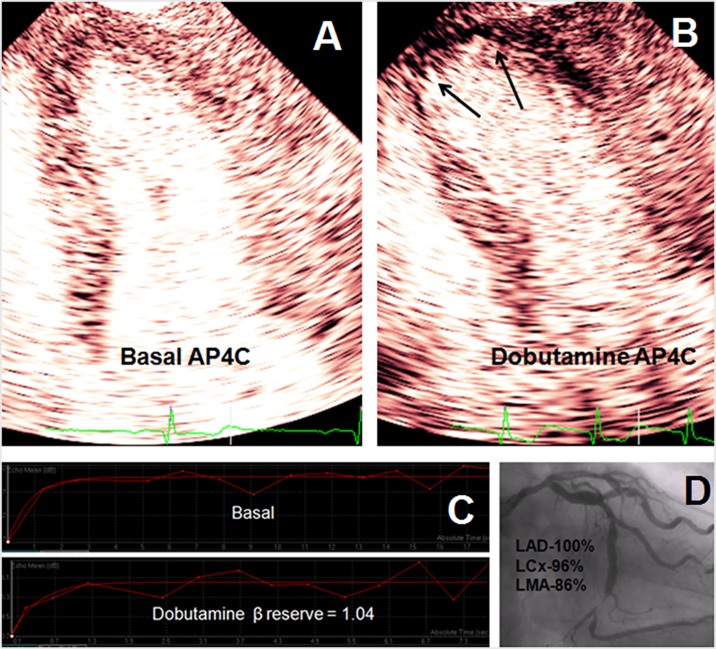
Apical four-chamber view imagin of a 67 year-old man with normal wall motion and qualitative myocardial perfusion at rest (A). (B) During dobutamine-stress, it was observed apical dyskinesis and marked perfusion defect (arrow). (C) Acoustic intensity curves at rest and during stress demonstrated a low β reserve. (D) Coronary angiography demonstrated significant coronary artery disease. Patient had event after 8 months of echocardiography. LAD-left anterior descending artery, LCx- left circumflex artery, LMA- left marginal artery.
